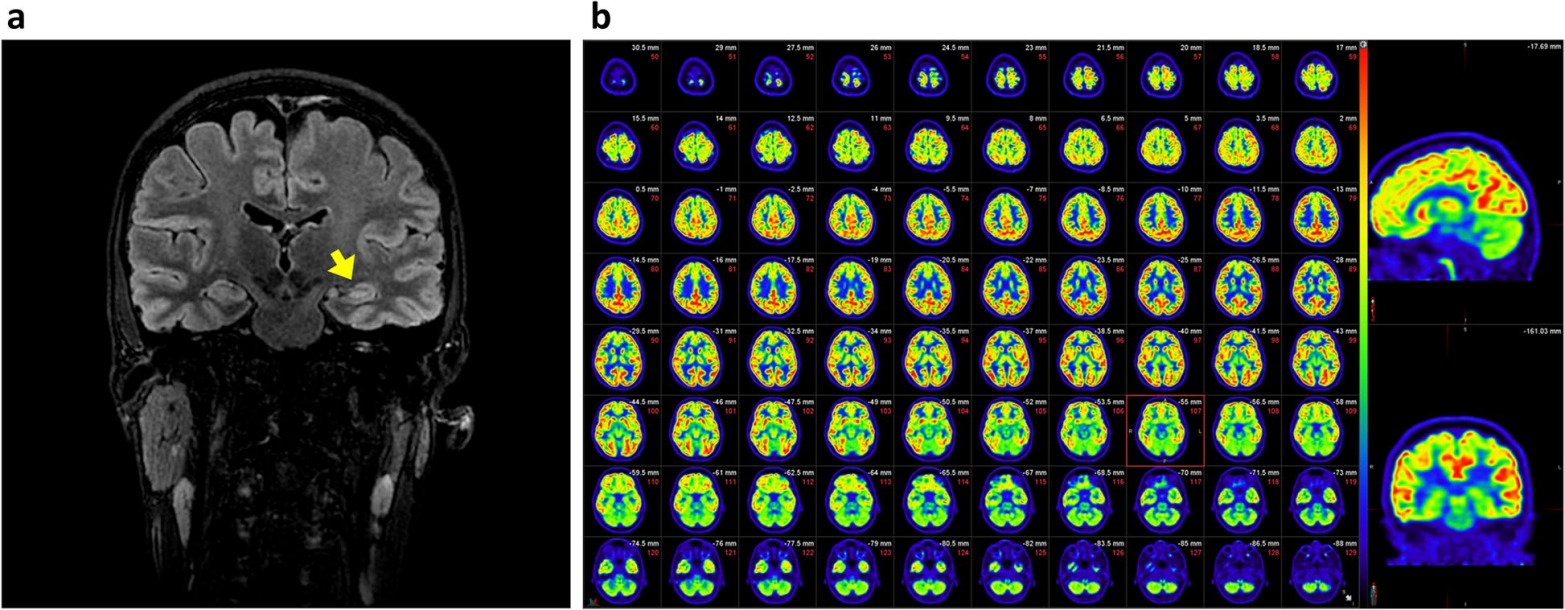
a Brain MRI showed left temporal and hippocampal sclerosis in the last follow up. Yellow arrow shows the increased T2/FLAIR signal and volume loss in the left hippocampus. b Brain PET showed no lateralized hypometabolism
